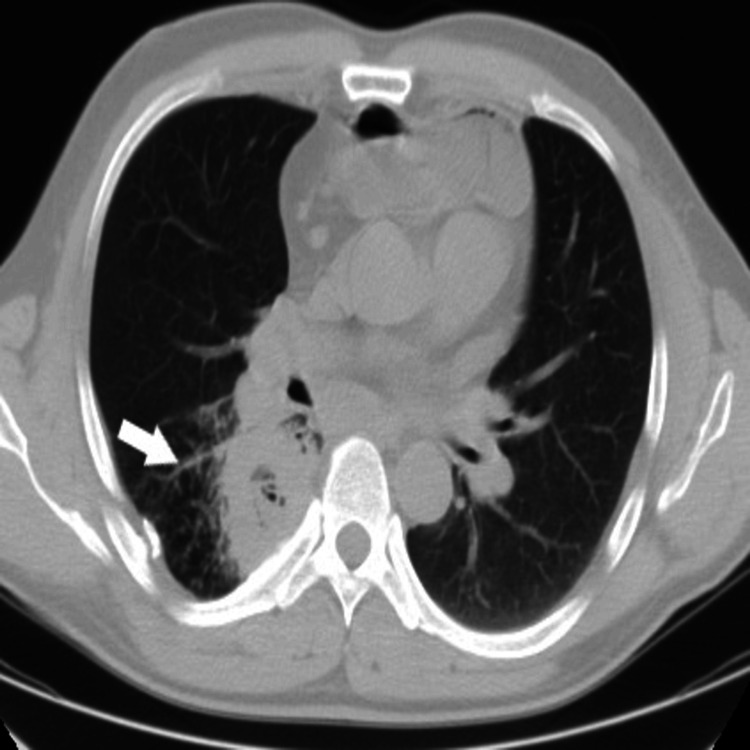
Computed tomography. Axial image of a right-sided lung abscess (white arrow).
